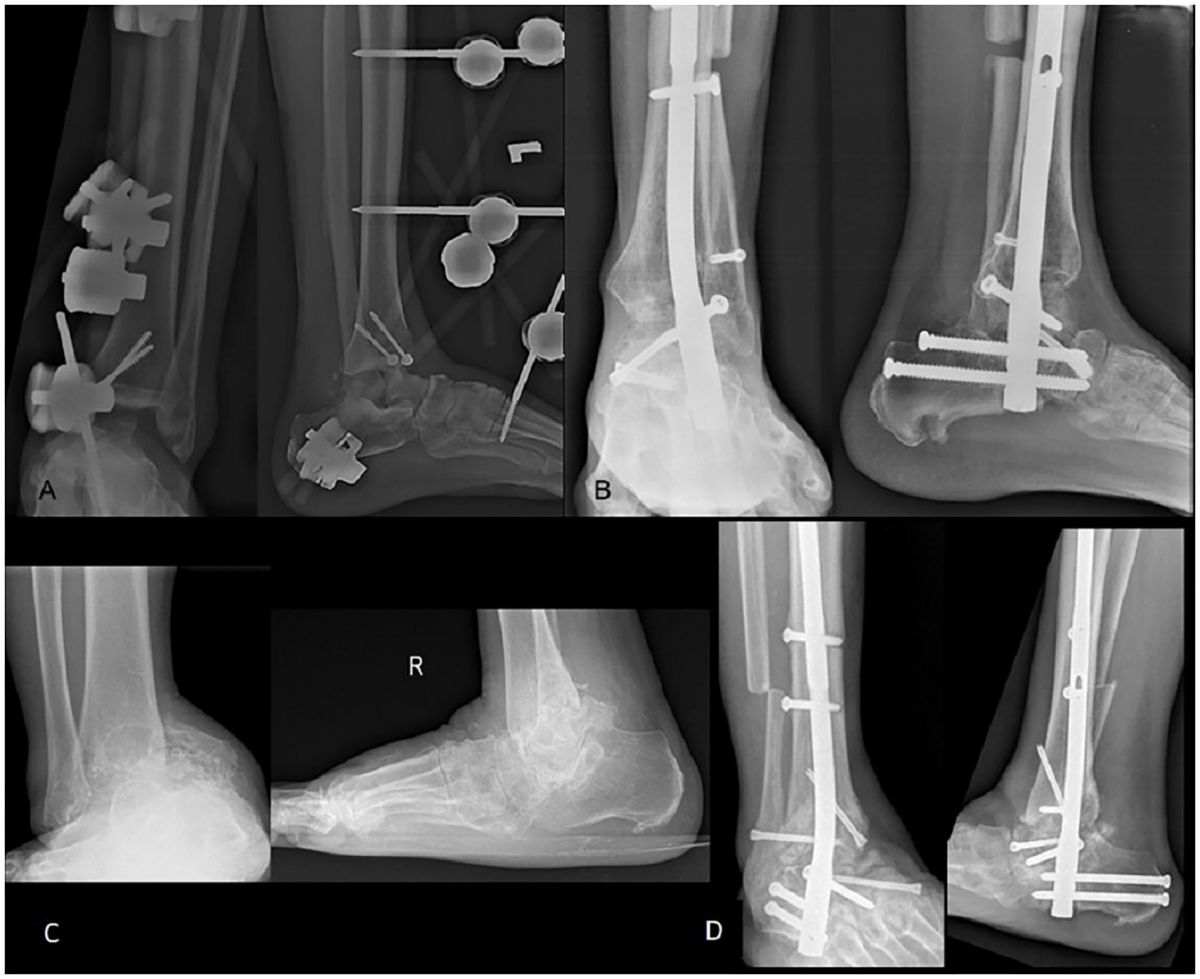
Radiograph of left tibia and fibula post ankle external fixator (A) and 1 year post ankle arthrodesis (B). Radiograph showing the destruction of the talus with ankle deformity (C). Ankle alignment was restored post ankle arthrodesis with femoral head allograft (D).
